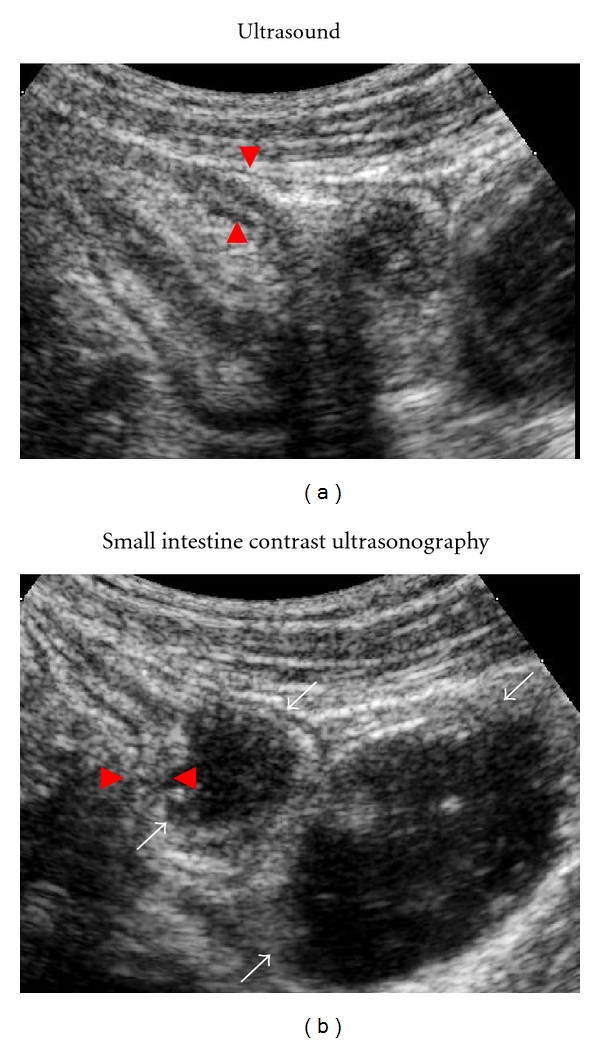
A 30-year-old male with stricturing Crohn's disease assessed by bowel ultrasound (without oral contrast) (a) and small intestine contrast ultrasonography (b). (a) shows Crohn's disease stenosis and prestenotic dilation, well defined in (b). Red arrowheads indicate bowel wall thickness in both panels, white arrows indicate prestenotic dilation in (b).
